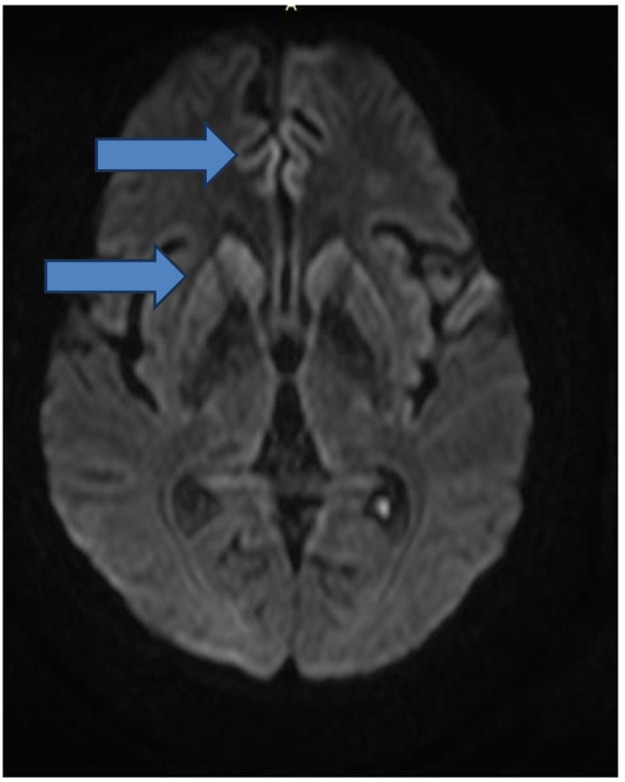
The brain MRI diffusion sequence images show a symmetric and bilateral hypersignal in the caudate and putamen, as well as in the superior frontal gyri (blue arrow).
